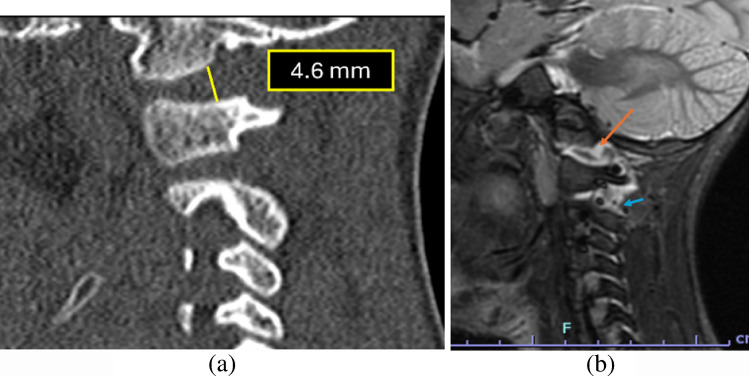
A 4-year-old child struck by a truck. (a) Sagittal CT of the cervical spine demonstrates an abnormally increased occipital condyle–C1 interval (CCI) on the right [N<2.5 mm] with a V-shaped widening of the joint suggestive of atlanto-occipital dissociation. (b) Subsequent MRI corroborates a distraction-type injury, showing fluid within the widened condyle–C1 joints (*orange arrow*) and adjacent soft-tissue edema, consistent with ligamentous disruption at the craniocervical junction. There is also fluid seen in the interspinous region at C1-C2 interval (*blue arrow*) indicative of soft-tissue injury
